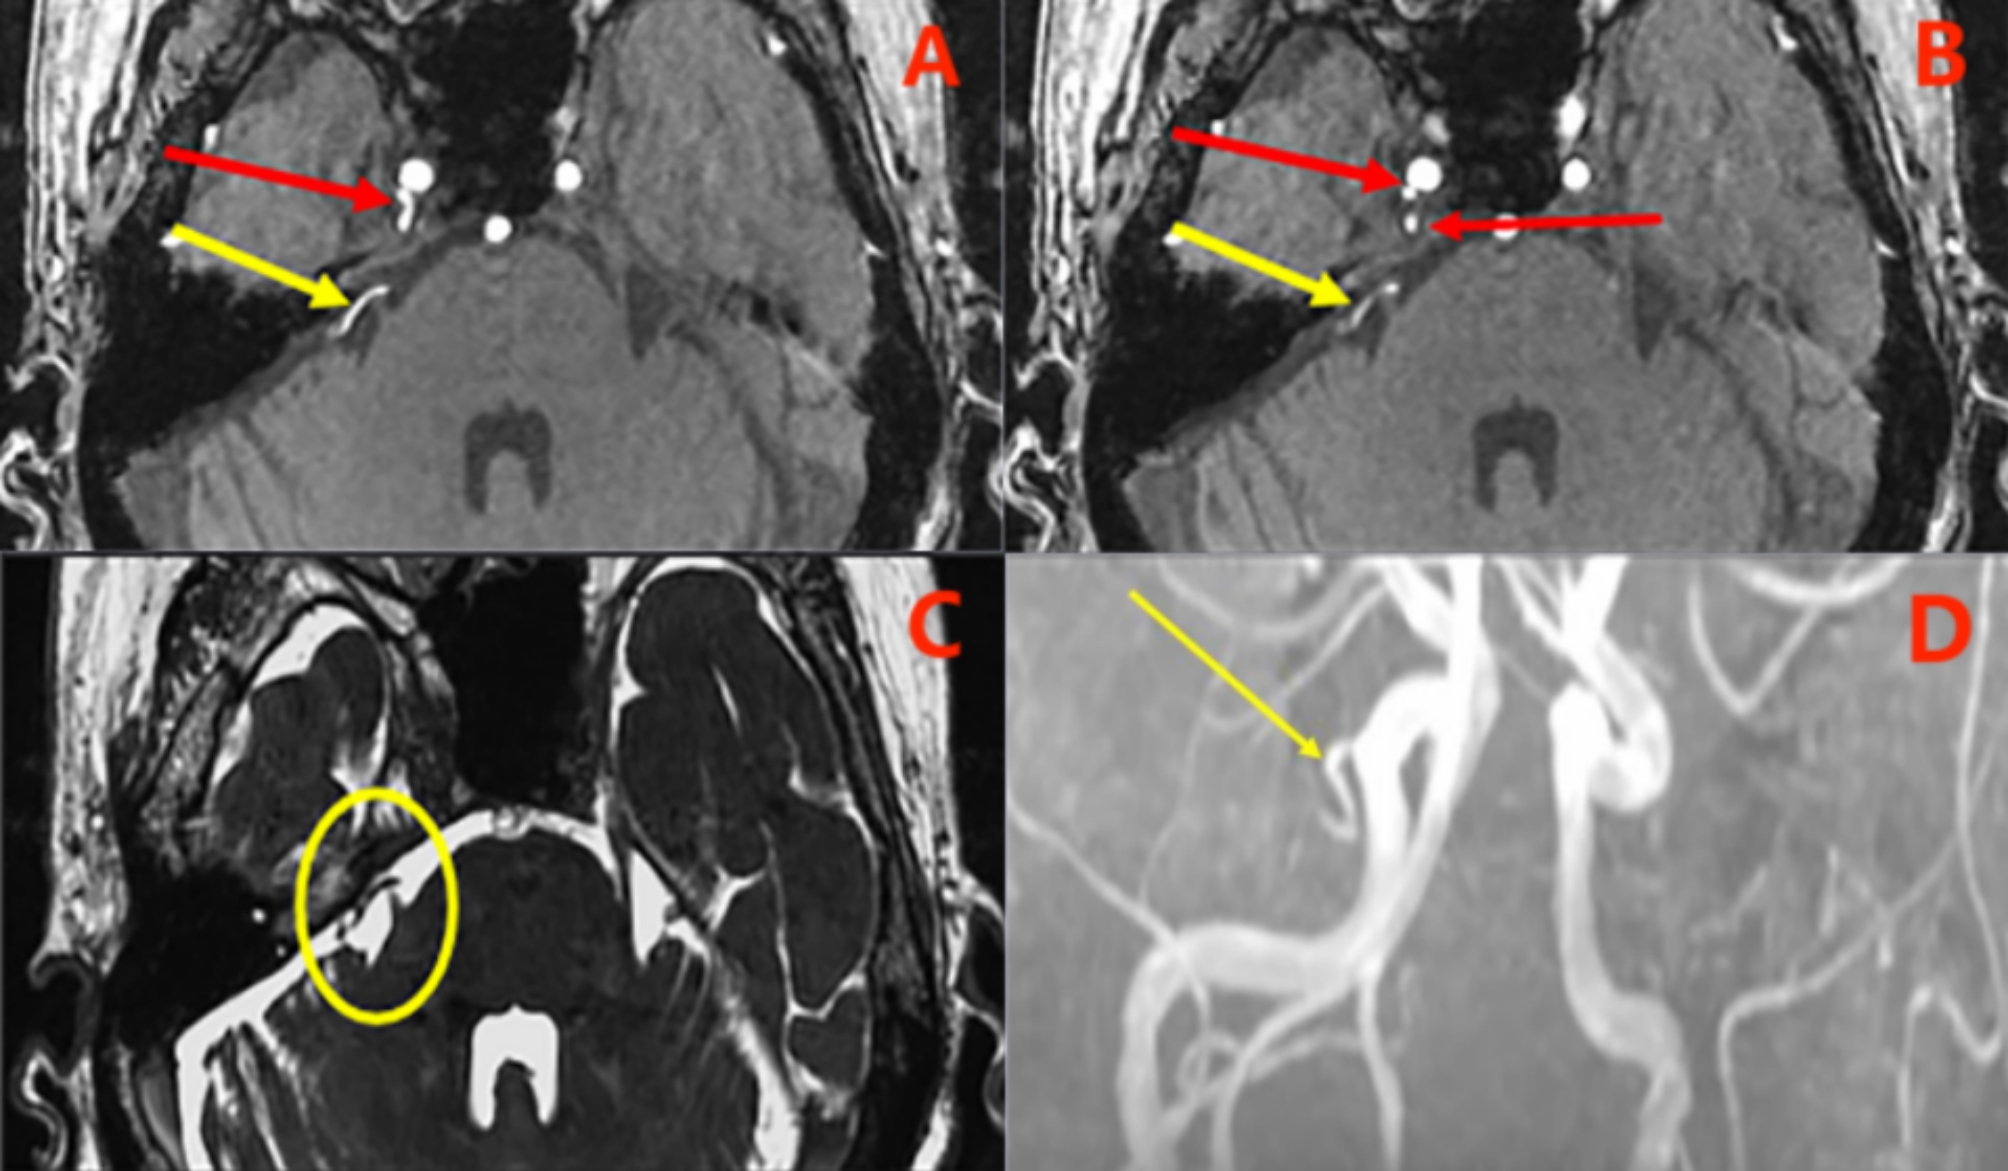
Preoperative magnetic resonance images. A&B, the original three-dimensional time-of-flight magnetic resonance angiography shows abnormal vessel compression in the cisternal segment of the right trigeminal nerve (yellow arrows). A persistent primitive trigeminal artery may originate from the right internal carotid artery (red arrows), which may enter Meckel’s cavity and be closely associated with the trigeminal nerve. C, three-dimensional fast imaging employing steady-state acquisition images show abnormal vessel compression in the cisternal segment of the right trigeminal nerve (yellow oval). D, preoperative maximum intensity projection reconstructed magnetic resonance angiography. A persistent primitive trigeminal artery (yellow arrow) originates from the precavernous portion of the right internal carotid artery
